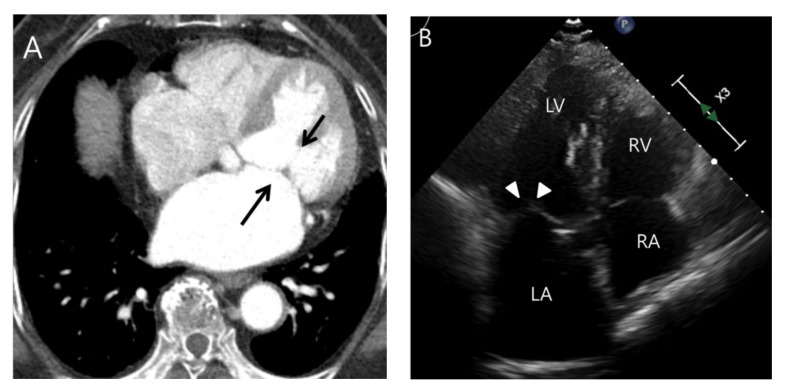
An example of a false positive diagnosis on routine chest CT due to motion artifact in an 84-year-old female patient. (A) Subtle nonelongated nodular thickening of the mitral valve is noted on an axial CT image (arrows). However, there was no splenic or renal infarction in this patient. (B) Subsequent echocardiography demonstrates negative findings (arrowheads).
